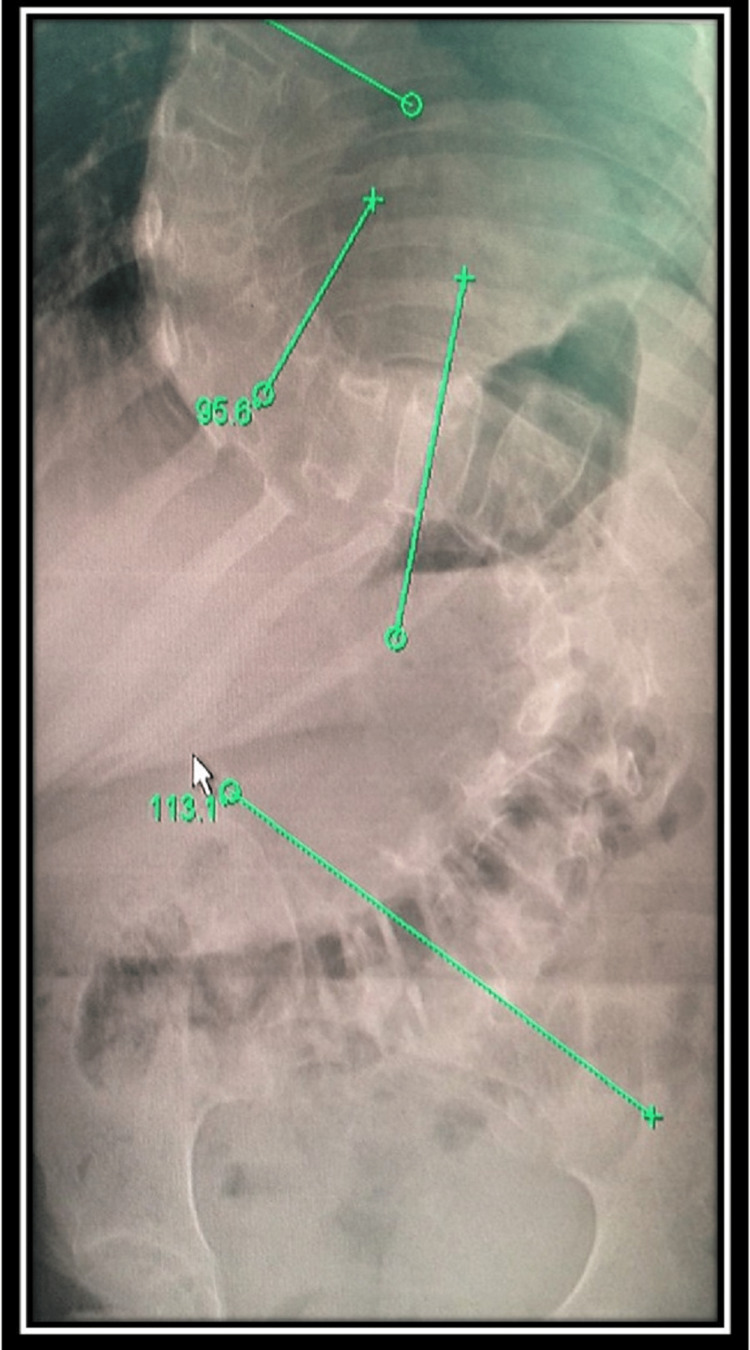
Pre-operative x-ray of the patient showing scoliosis with Cobb’s angle.
